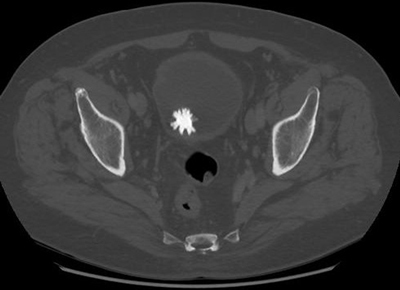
Axial non-contrast CT scan in supine position. A spiculated bladders stone, or ‘Jackstone’, is seen posteriorly in the bladder.
